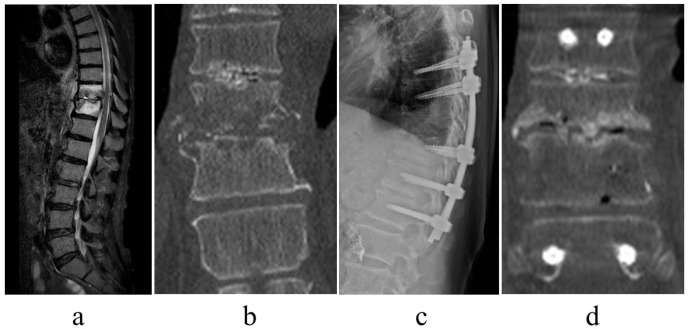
Images of a representative case of osteosclerosis of the endplate. A 71-year-old man, dialysis because of chronic renal failure, T10/11 pyogenic spondylitis. Short tau inversion recovery of magnetic resonance imaging revealed high signal changes in the vertebral body ((a), sagittal view), and computed tomography (CT) demonstrated significant bone destruction of the endplate((b), coronal view). The patient experienced persistent pain and underwent the posterior fixation of T8–L1 with percutaneous pedicle screw ((c), plain radiograph of thoracolumbar lateral view). One year postoperatively, the vertebral body was slightly crushed, and the endplate was osteosclerotic ((d), coronal view of CT). The patient exhibited improvement without recurrence of infection.
